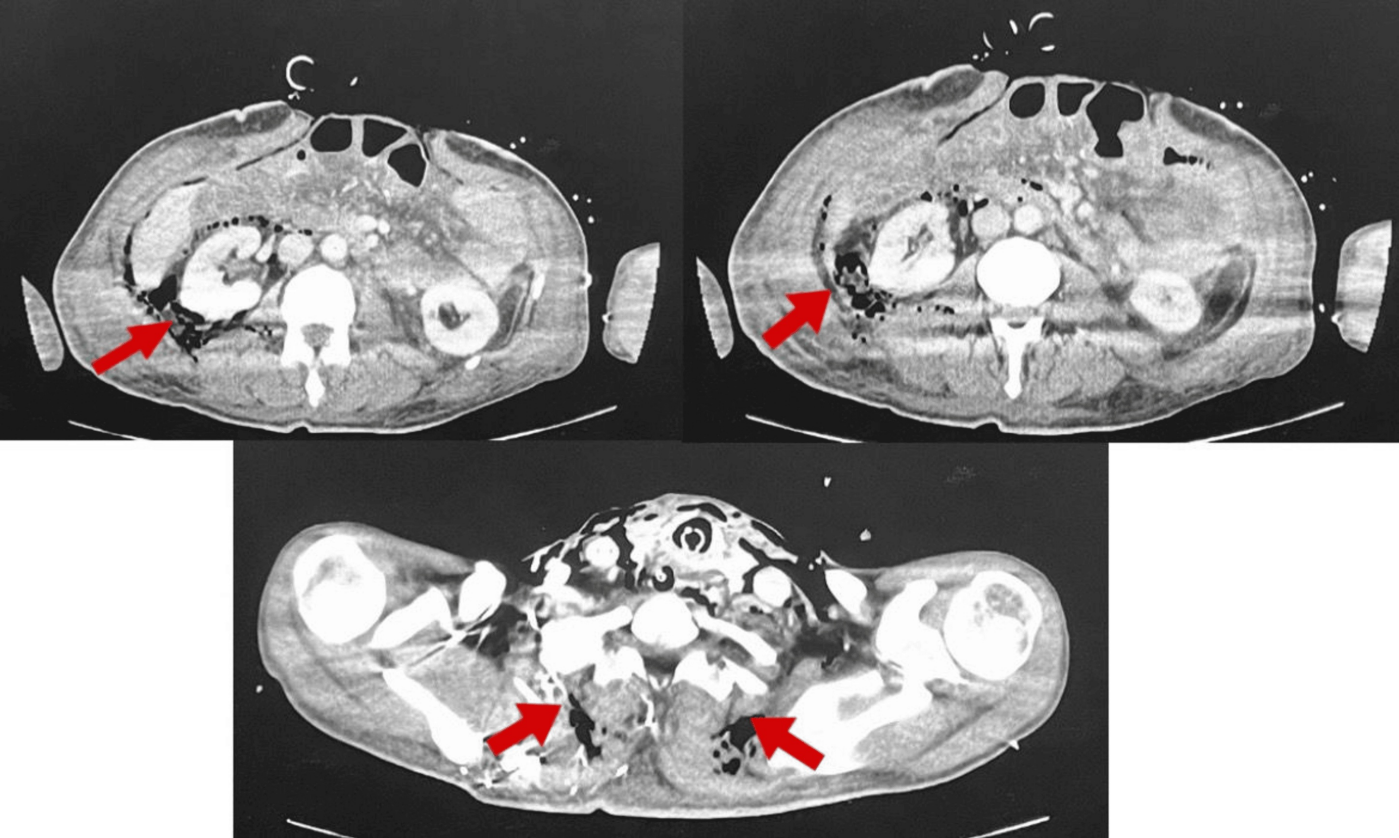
Computed tomography of the thorax, abdomen, and pelvis done on May 5, 2025, showed a significant reduction in the pneumomediastinum, surgical emphysema, and pneumoretroperitoneum
